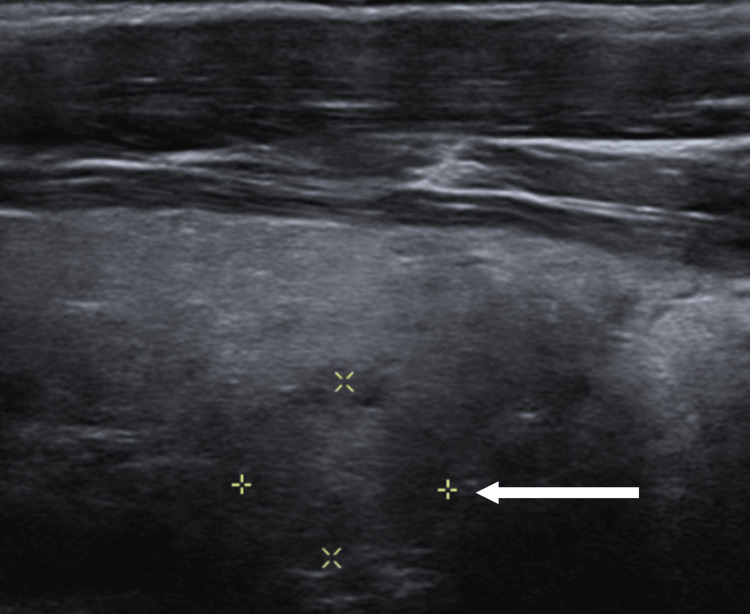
Neck imaging. Neck ultrasound showing a 1.2 x 1 x 1 cm left nodule (arrow), interpreted as a possible parathyroid adenoma.
